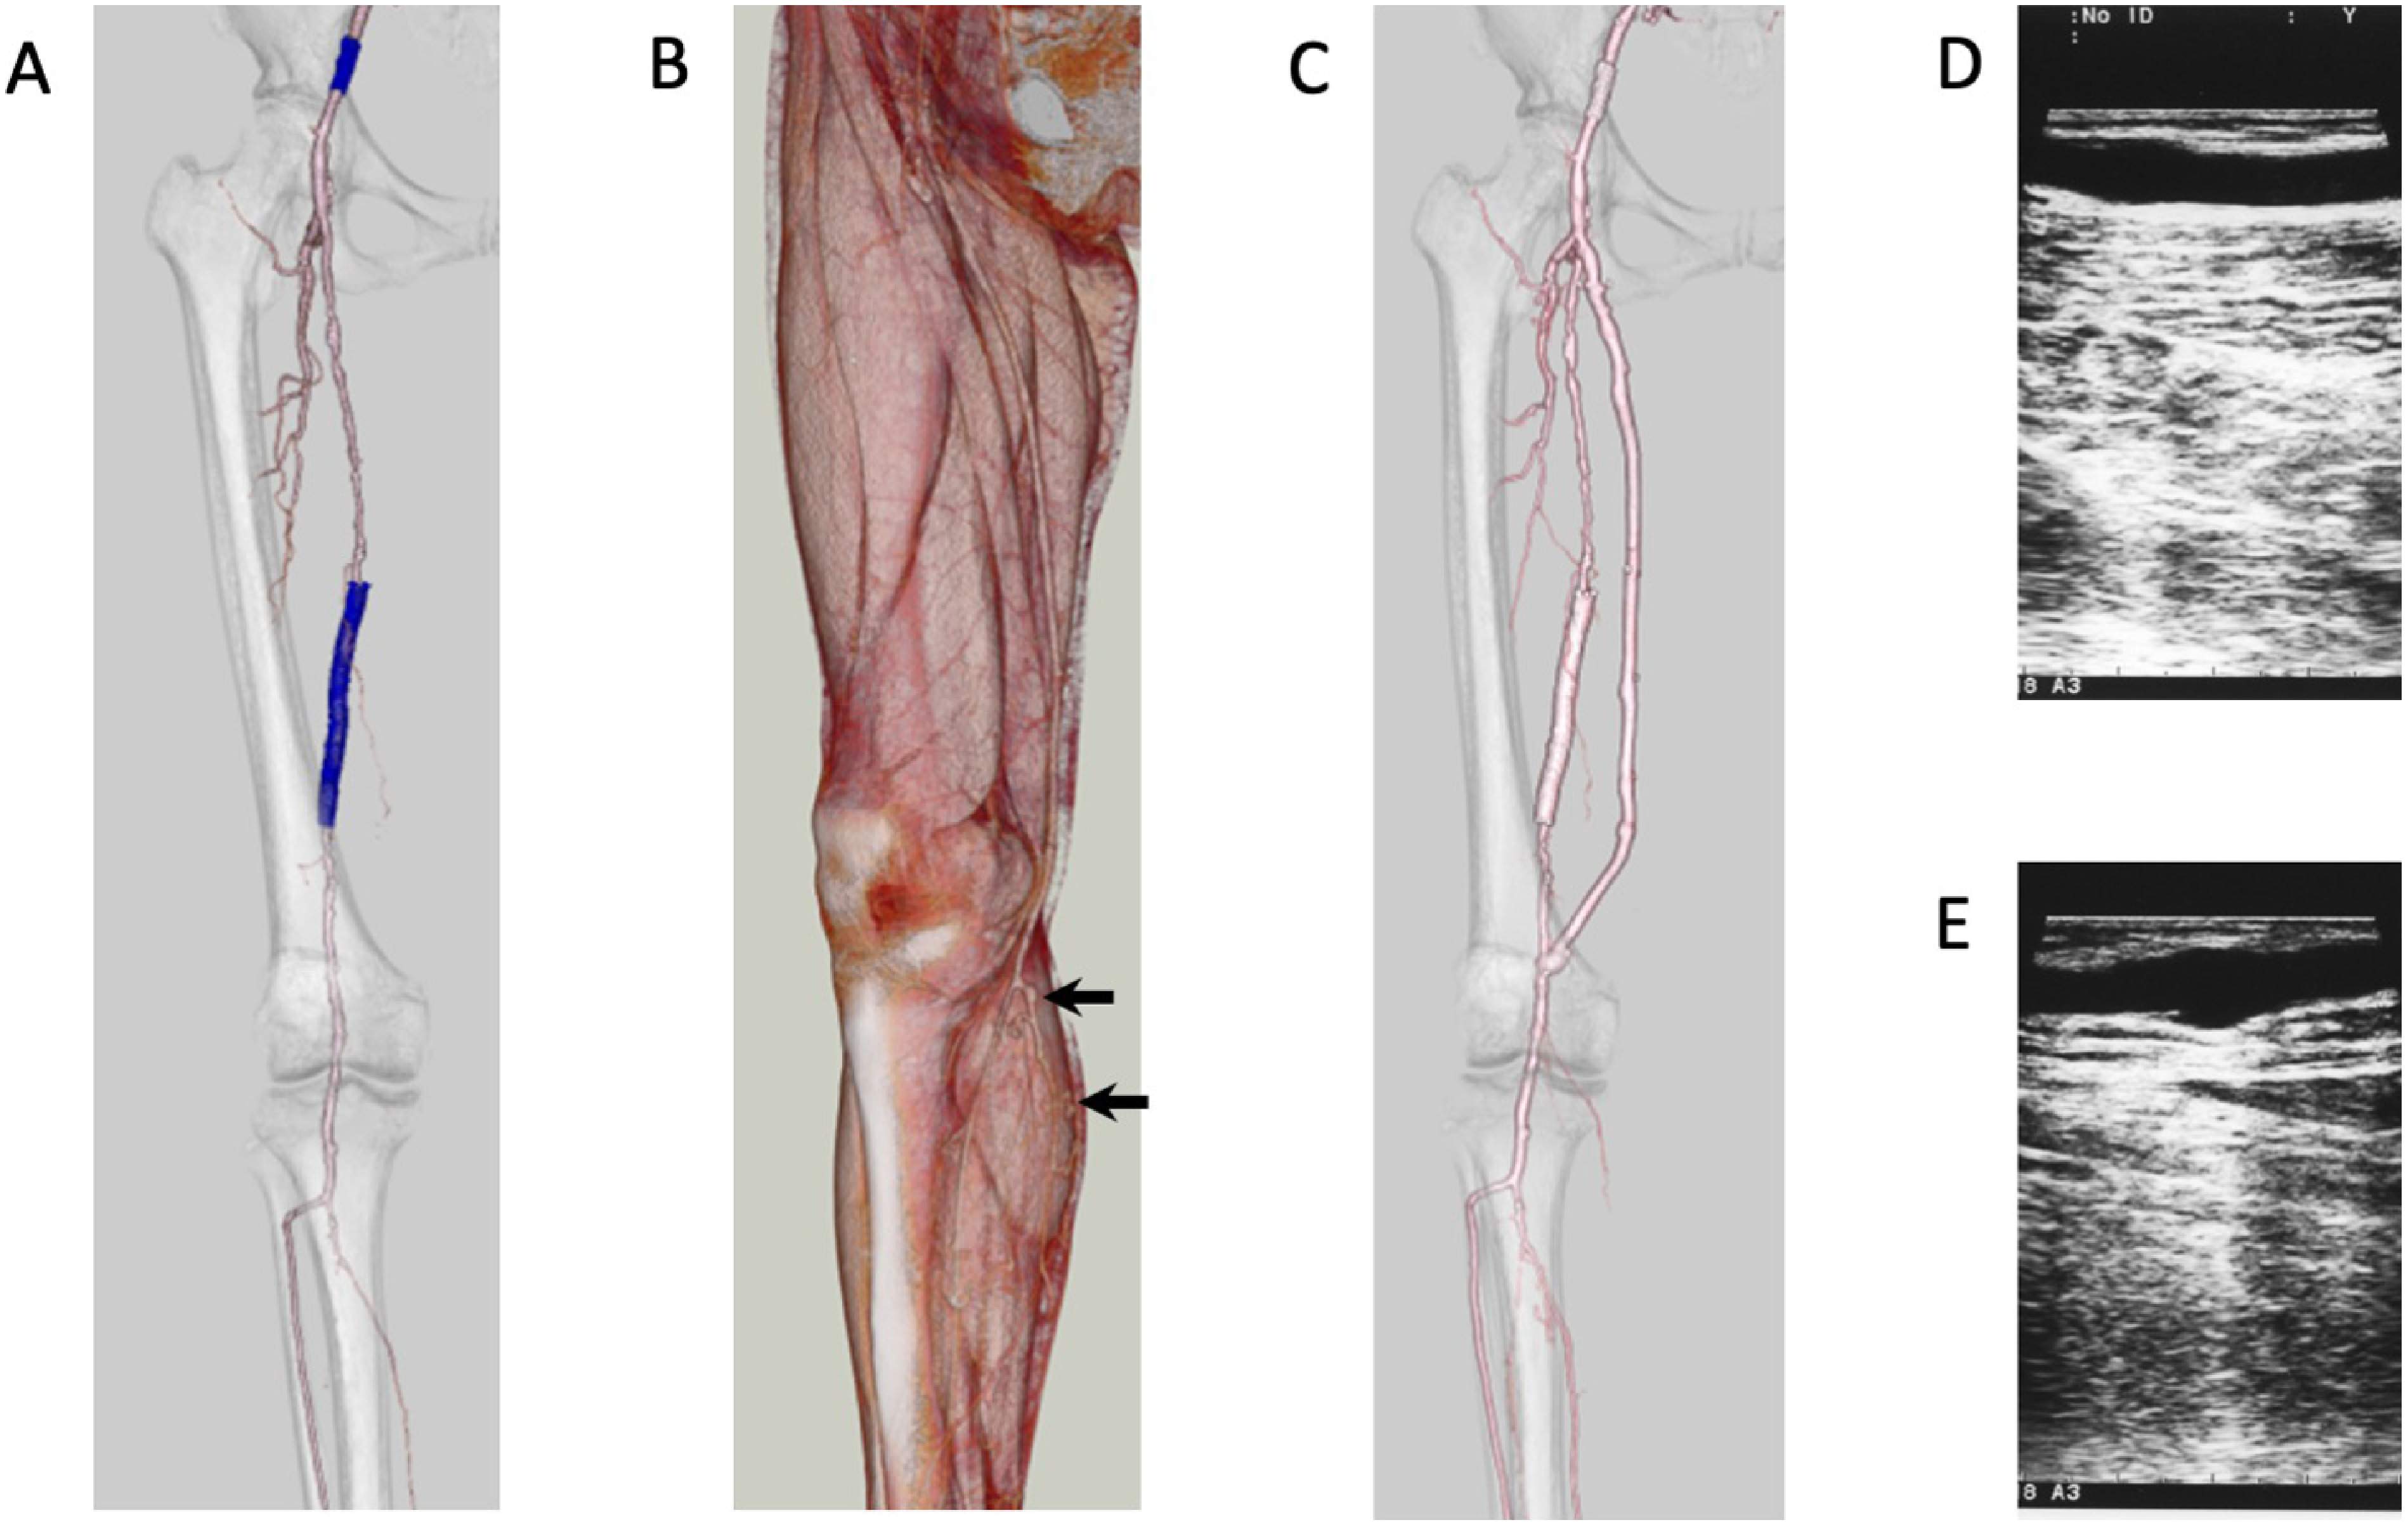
Fig. 1 Case 1. (A) Preoperative computed tomography angiography revealed the patency of the right iliac and popliteal arteries as well as stenosis of the right superficial femoral artery stent. (B) Preoperative computed tomography (CT) venography revealed the great saphenous vein having no morphologically conspicuous abnormalities except for some segmental type varicosity at the crus (arrows) and some sinusoid dilatations at the origin of valves. (C) Postoperative CT angiography revealed that the right femoropopliteal bypass was patent. (D) Duplex ultrasonography (DUS) showed no structural changes in the vein graft. (E) DUS showed some sinusoid dilatations at the origin of the valves.
